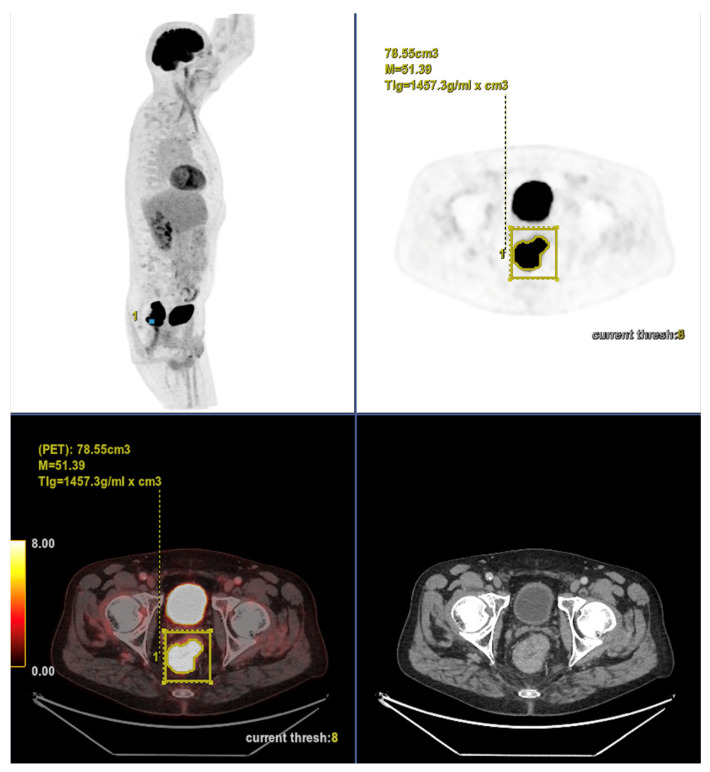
Representative example of manual delineation of a primary rectal tumor on baseline FDG PET/CT showing manual delineation of the metabolically active tumor volume (first row) on the PET image with corresponding fused PET/CT image and CT image with iodinated contrast medium (second row). Morphological features, including tumor shape (circular or semi-circular), cranio-caudal extension (in cm), and overall tumor volume (in cm^3^), were assessed on the CT image (often with contrast medium) coregistered with the PET images. Tumor shape was determined on the axial plane, cranio-caudal extension on the sagittal plane, and tumor volume after careful delineation on all planes. Iodinated contrast medium was administrated in 70% of cases after exclusion of contraindications. Metabolic parameters, including SUVmax, SUVmean, MTV and TLG, were systematically extracted from the segmented tumor (yellow in this figure). SUVmax (M in this figure) and TLG were automatically displayed. SUVmean could be obtained by simply replacing SUVmax with SUVmean in the parameter selection. MTV was automatically calculated from TLG and SUVmean values displayed on the workstation. The highlighted region in yellow represents the 3D contour of the primary tumor, excluding physiological uptake and non-tumor regions.
